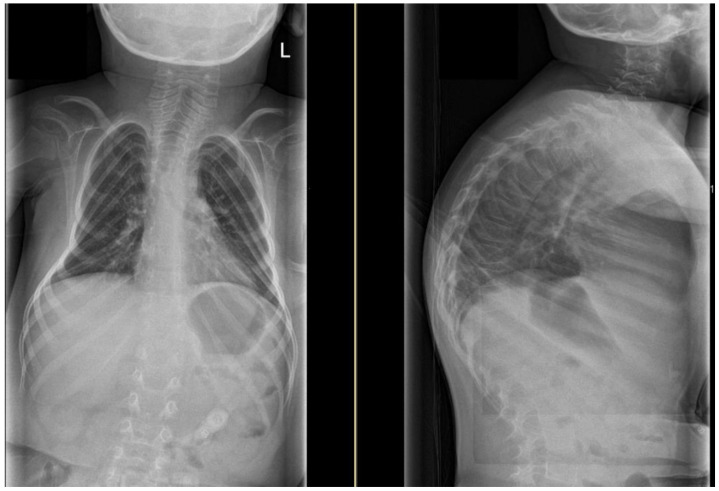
Anteroposterior and lateral sitting radiographs of patient no. 13.
